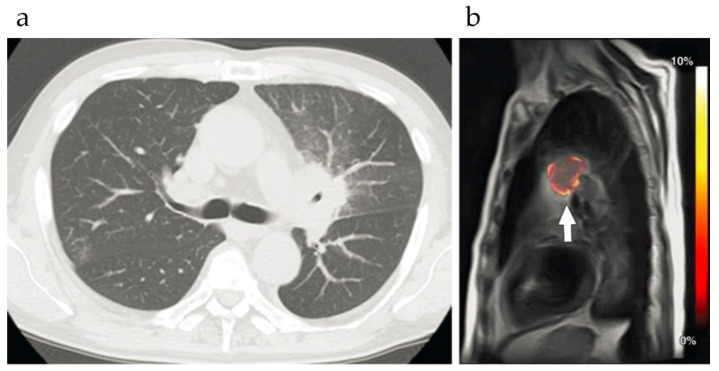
Squamous cell carcinoma in a 66 year-old man. (a) Axial thin-section CT image (lung window setting) shows a left hilar mass, with obstructive pneumonia. (b) Sagittal APT-weighted CEST MR map shows a left hilar mass (arrow) with low MTR_asym_ (at 3.5 ppm) and relatively high MTR_asym_ (at 3.5 ppm) of the surrounding obstructive pneumonia. Reproduced with permission from Radiological Society of North America 2016 (DOI: 10.1148/radiol.2015151161) [82].
